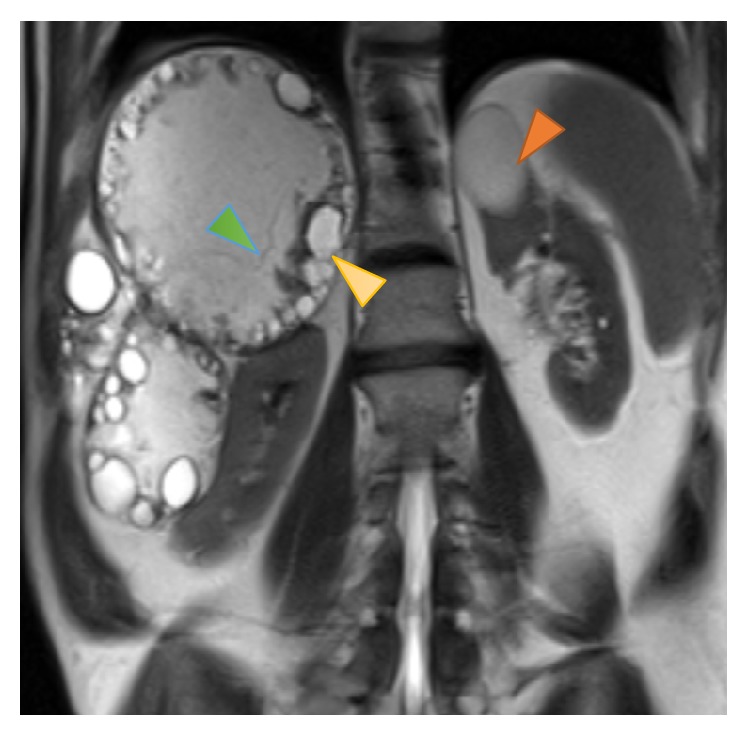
Coronal T2-weighted MR image shows multiple abdominal hyperintense lesions with smaller peripheral cysts compatible with daughter cysts (yellow arrow) and also thin linear septation, compatible with the detachment of the laminated membrane of the pericyst (green arrowhead). Note also a simple renal cyst (orange arrowhead), probably an incidental finding.
